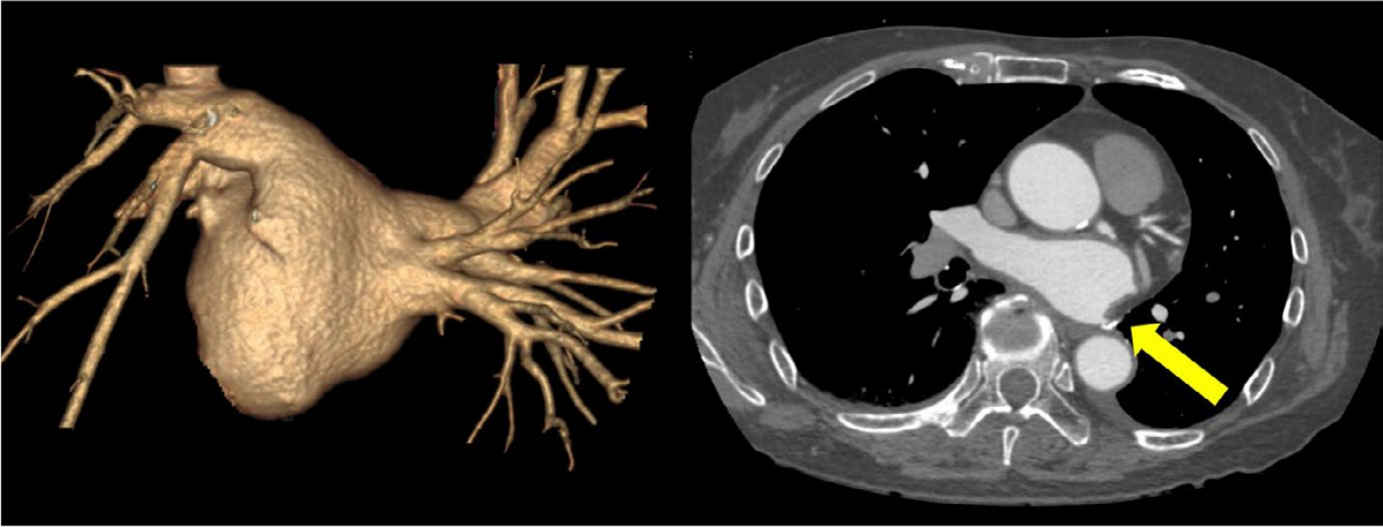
Case 1 had undergone left lower lobectomy, resulting in the left inferior PV being transected. Contrast‐enhanced CT shows that the left inferior PV was the PV stump (yellow arrow).
